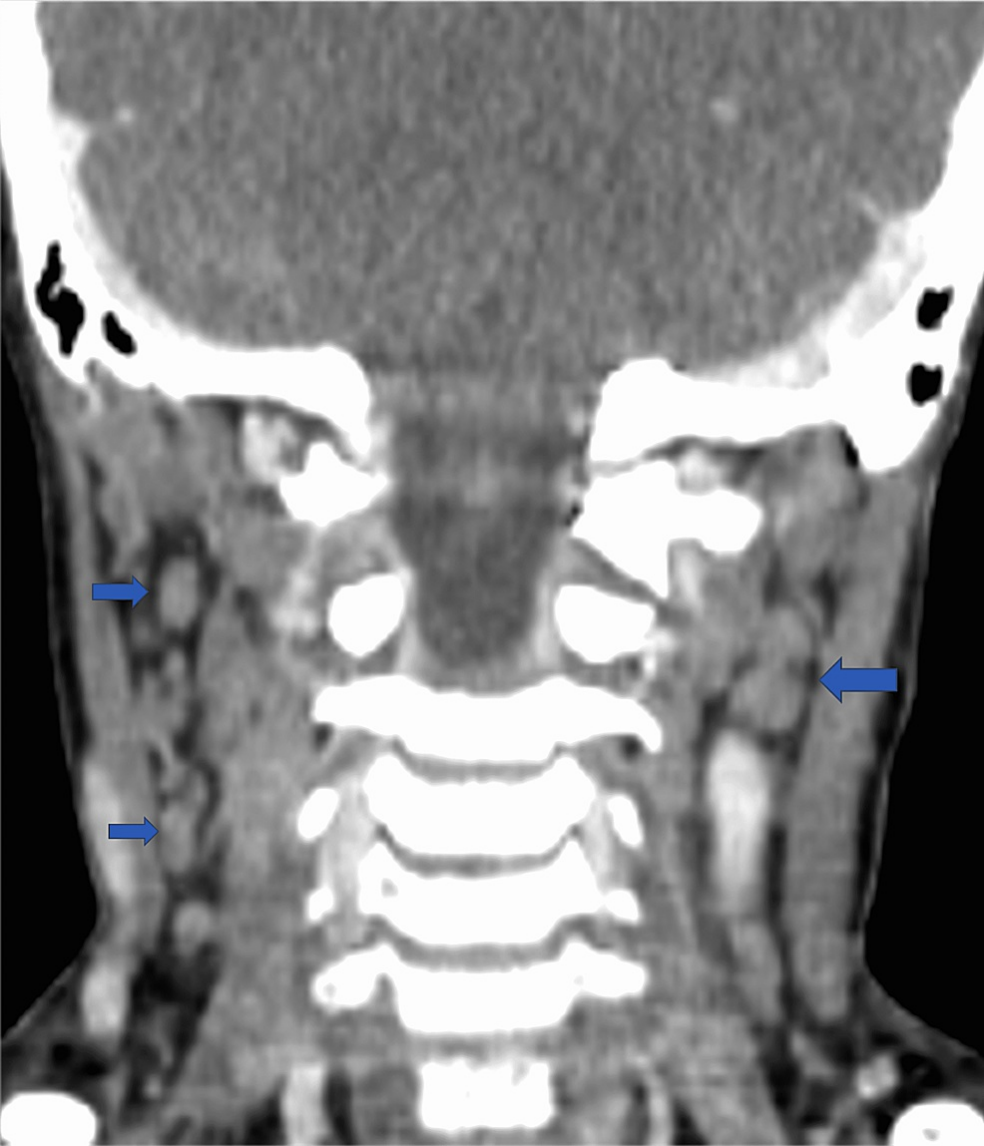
Sagittal CT scan of the neck showing multiple bilateral cervical lymphadenopathies (blue arrows).
